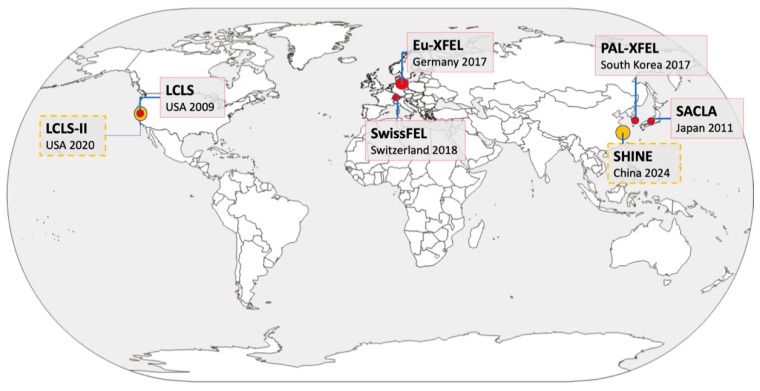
A map of X-ray free electron laser (XFEL) facilities. Five of these are in operation (red circles), and two facilities (Linac Coherent Light Source (LCLS)-II and Shanghai HIgh repetition rate XFEL aNd Extreme light facility (SHINE), orange circles) are designed to operate at 1 MHz in continuous wave (CW) mode, in contrast to the long pulse (LP) mode in Eu-XFEL.
